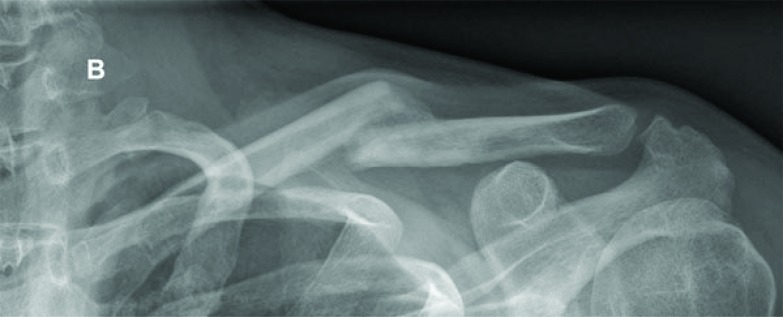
The antero-posterior radiograph of the same fracture at follow-up 16 weeks after trauma demonstrates an only marginal increase of the initial displacement with progressive callus formation.
